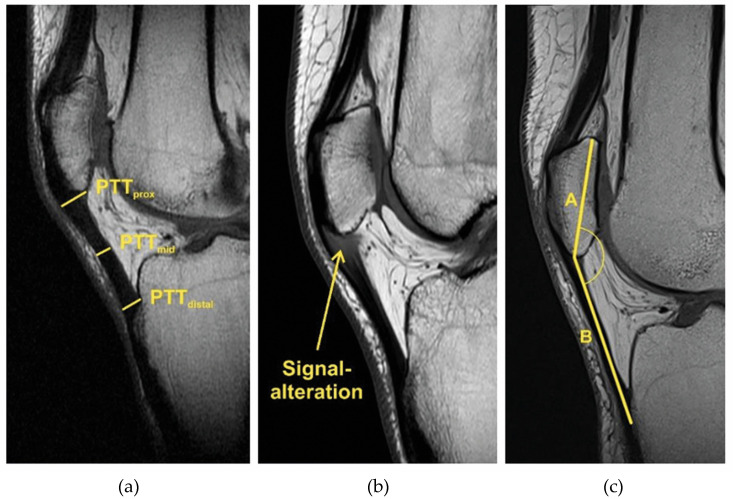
(a) Patellar tendon thickness (PTT): shown here on MRI, a thickened patellar tendon with PT in the proximal (PTTprox), middle (PTTmid) and distal segments (PTTdistal). (b) Signal intensity (alteration) in PT at the origin of the patellar tendon. (c) Patella–patellar tendon angle (PPTA): angle between the line drawn from the base of the patella to the apex (A) and the line drawn between the apex of the patella to the insertion of the patellar tendon on the tibial tuberosity (B).
